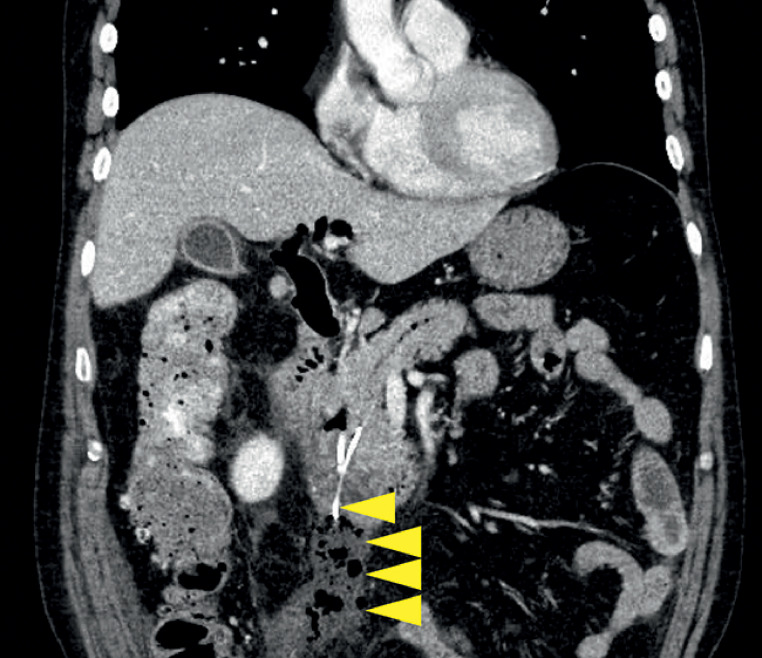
Computed tomography image showing evidence of duodenal perforation due to stent deviation (arrow heads).
